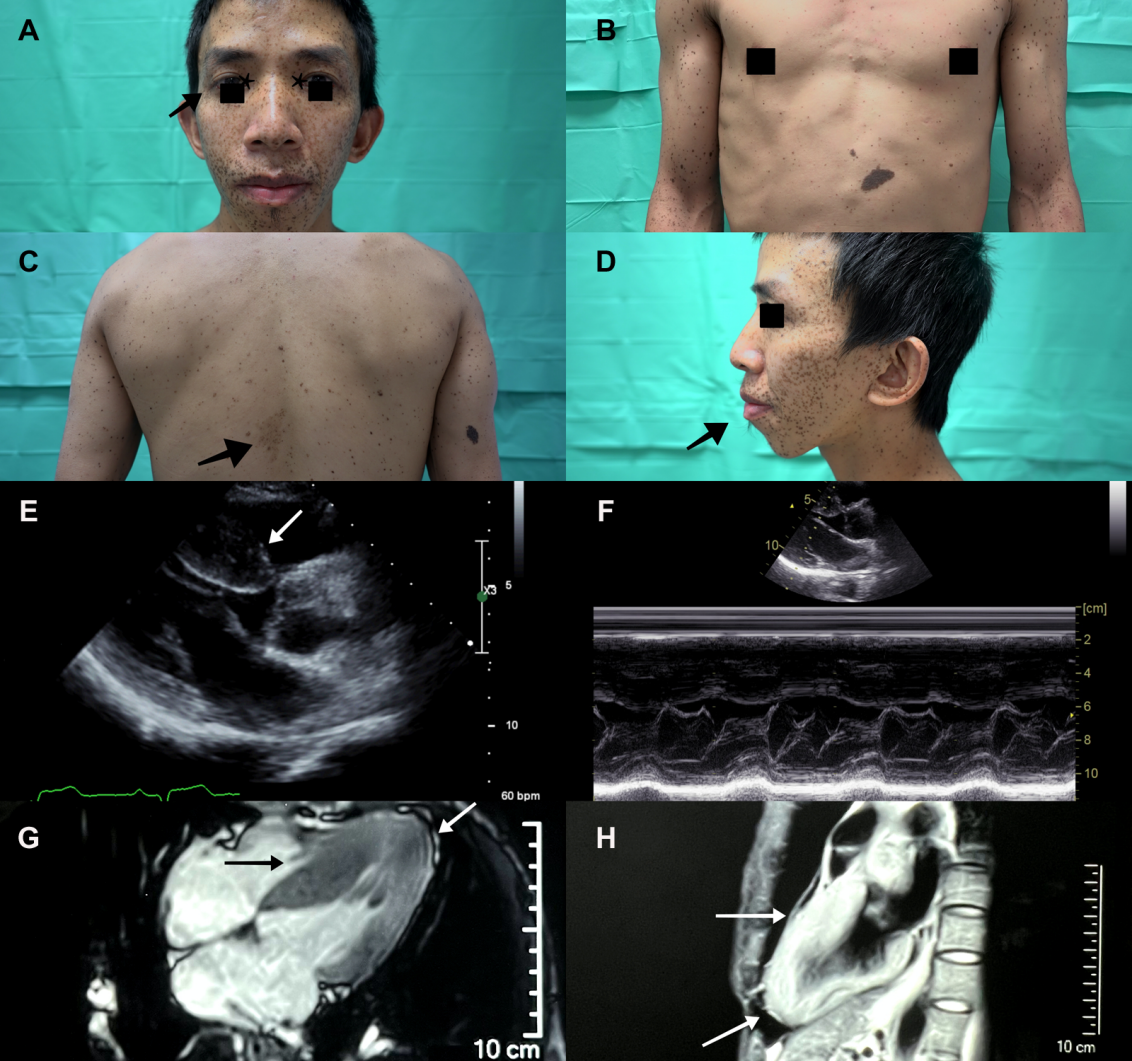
A Diffuse black to brownish lentigines seen mainly on the face of the proband (individual II-3). Ocular hypertelorism (asterisk), ptosis (arrowhead) and thick lips were remarkable. (B) Multiple lentigines over the anterior trunk and limbs varied in shape and size, and with diameters ranging from to 5 mm. (C) A café au lait macule (arrowhead) was seen on his mid-back. (D) Low set ears and retrognathism (arrowhead) were revealed. Echocardiography demonstrated (E) eccentric left ventricular hypertrophy (septal wall thickness: 22 mm, arrowhead), (F) without left ventricle outflow tract obstruction. (G,H) Cardiac magnetic resonance imaging showed hypertrophic segments over septal and apical walls (arrowhead).
